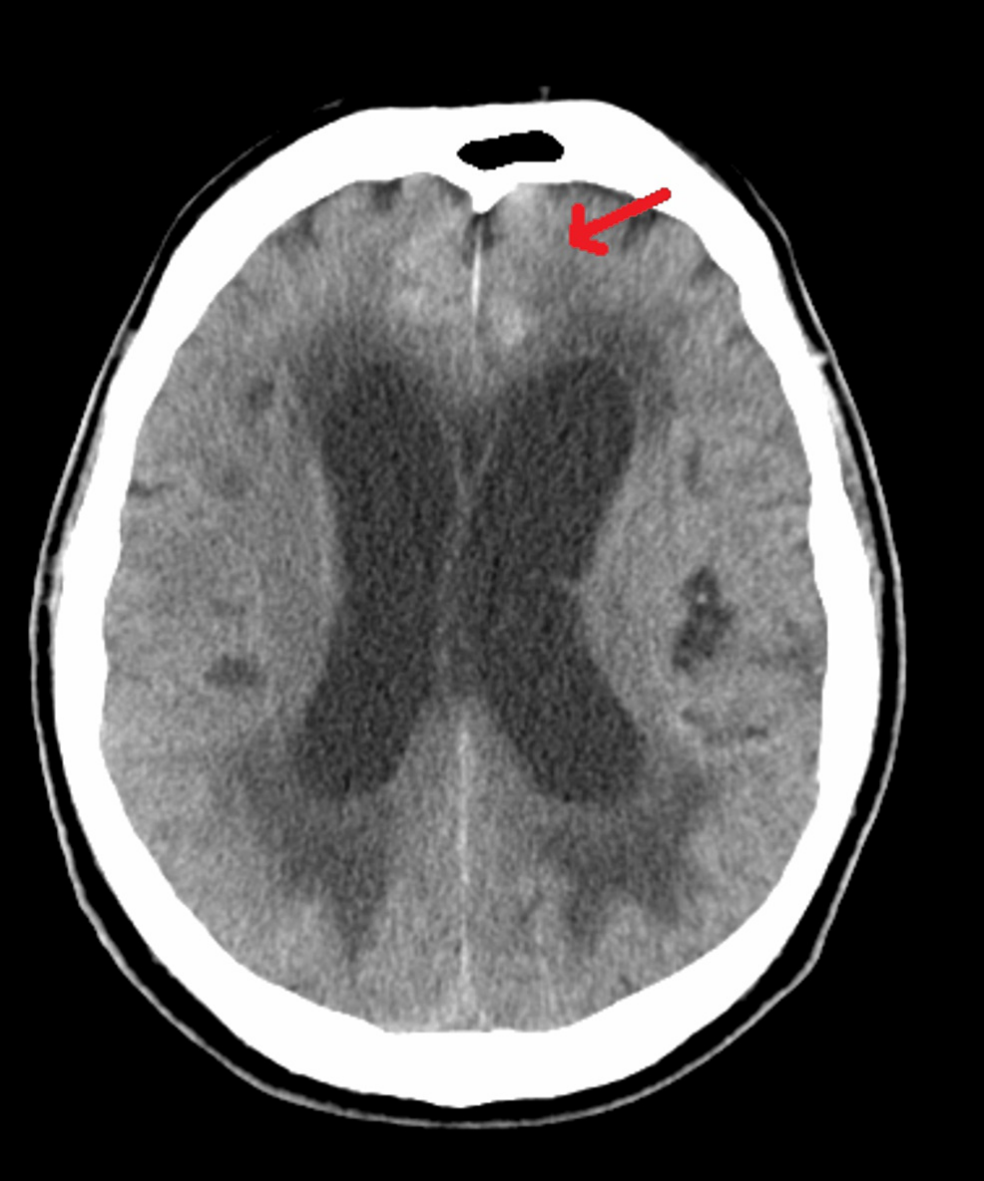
CT of the head without contrast showing subarachnoid hemorrhage above the cortical sulci of the bifrontal lobes with intraventricular extension as well as an interval increase in the hydrocephalus.
